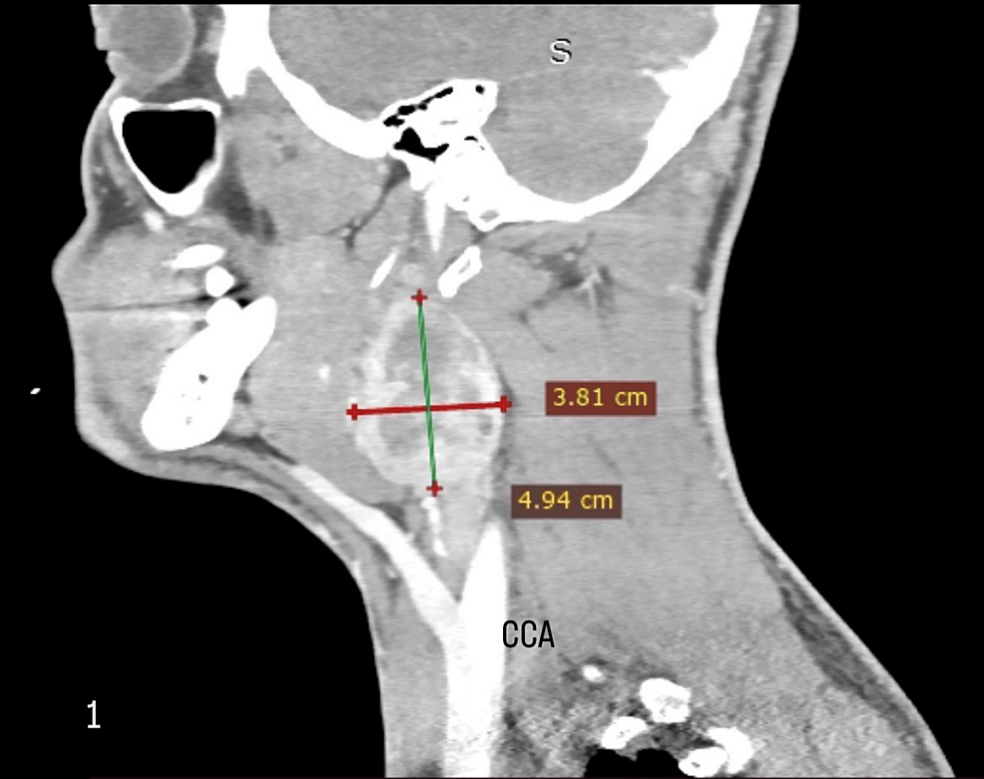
Computed tomography angiography of the neck shows an oval-shaped, well-defined mass located in the left carotid bifurcation, measuring 3.8 cm x 4.94 cm, highly enhanced by the contrast agent. CCA: common carotid artery
